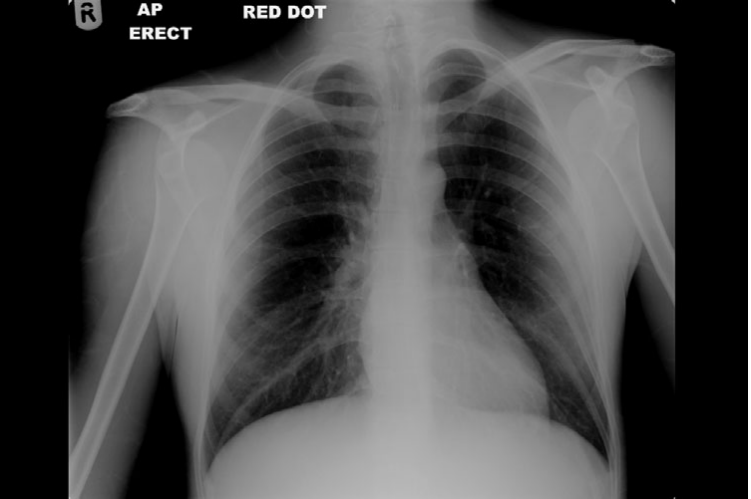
An AP radiograph demonstrating bilateral anterior shoulder dislocations.
